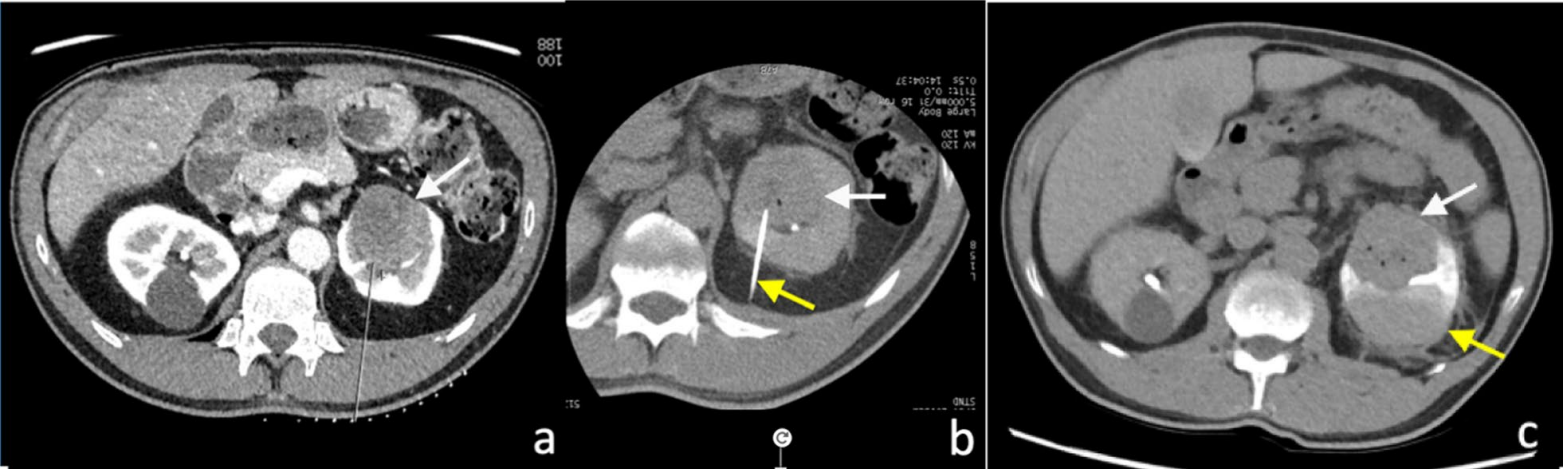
a Axial CT image pre-biopsy planning image of percutaneous posterior approach of a left renal cell carcinoma (white arrow) and (b) image demonstrating a coaxial biopsy needle system (yellow arrow) within the left renal cell carcinoma (white arrow). c Subcapsular haematoma (yellow arrow) post-percutaneous biopsy of the left anterior renal cell carcinoma (white arrow). The 18-gauge core biopsies confirmed the diagnosis of mucinous tubular and spindle cell renal cell carcinoma
